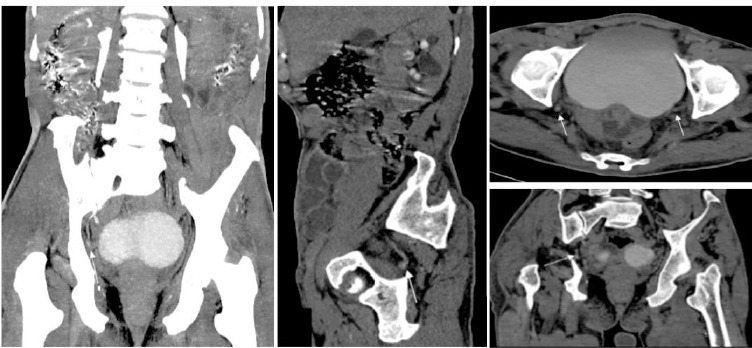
Computed tomography* (CT) image showing the normal ureteric course in preoperative images
